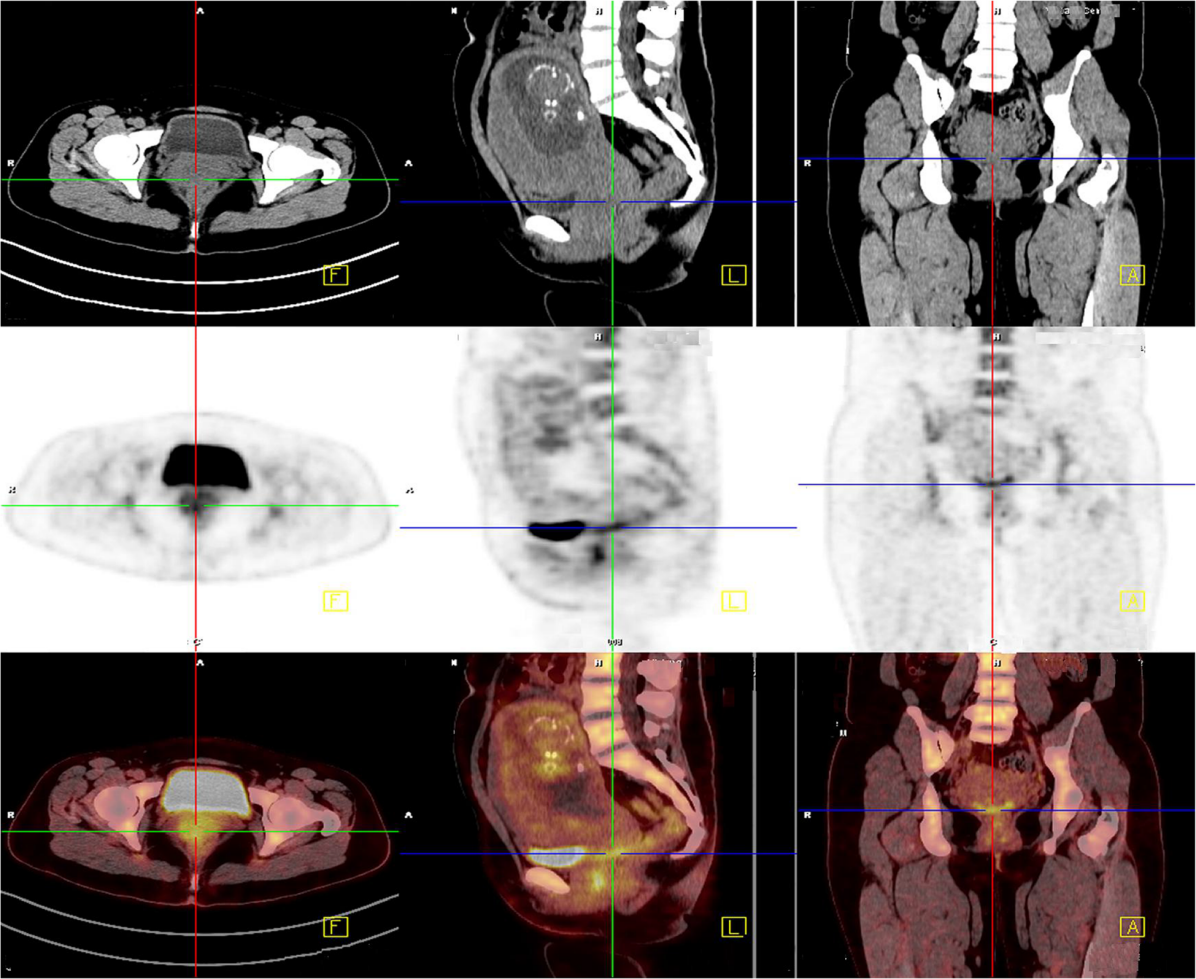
PET-CT examination revealed that the patient’s cervix was thickened and that her metabolism was increased. No obvious tumor was seen in other areas
